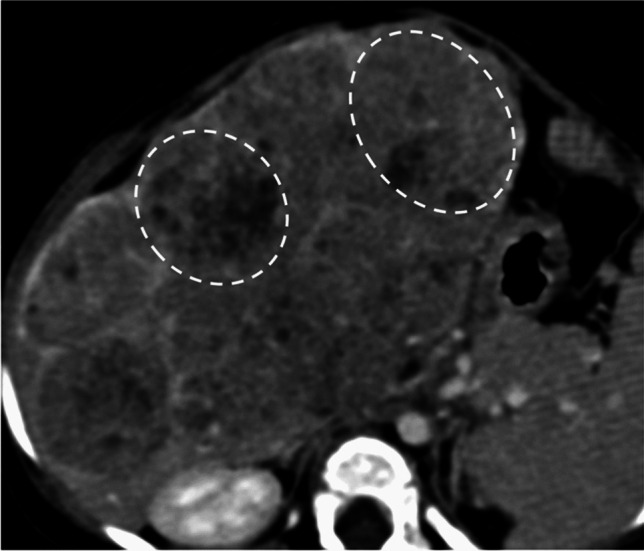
Axial portal venous phase abdominal CT in a 12-month-old girl with hepatoblastoma demonstrating its lobulated morphology (dashed circles)
